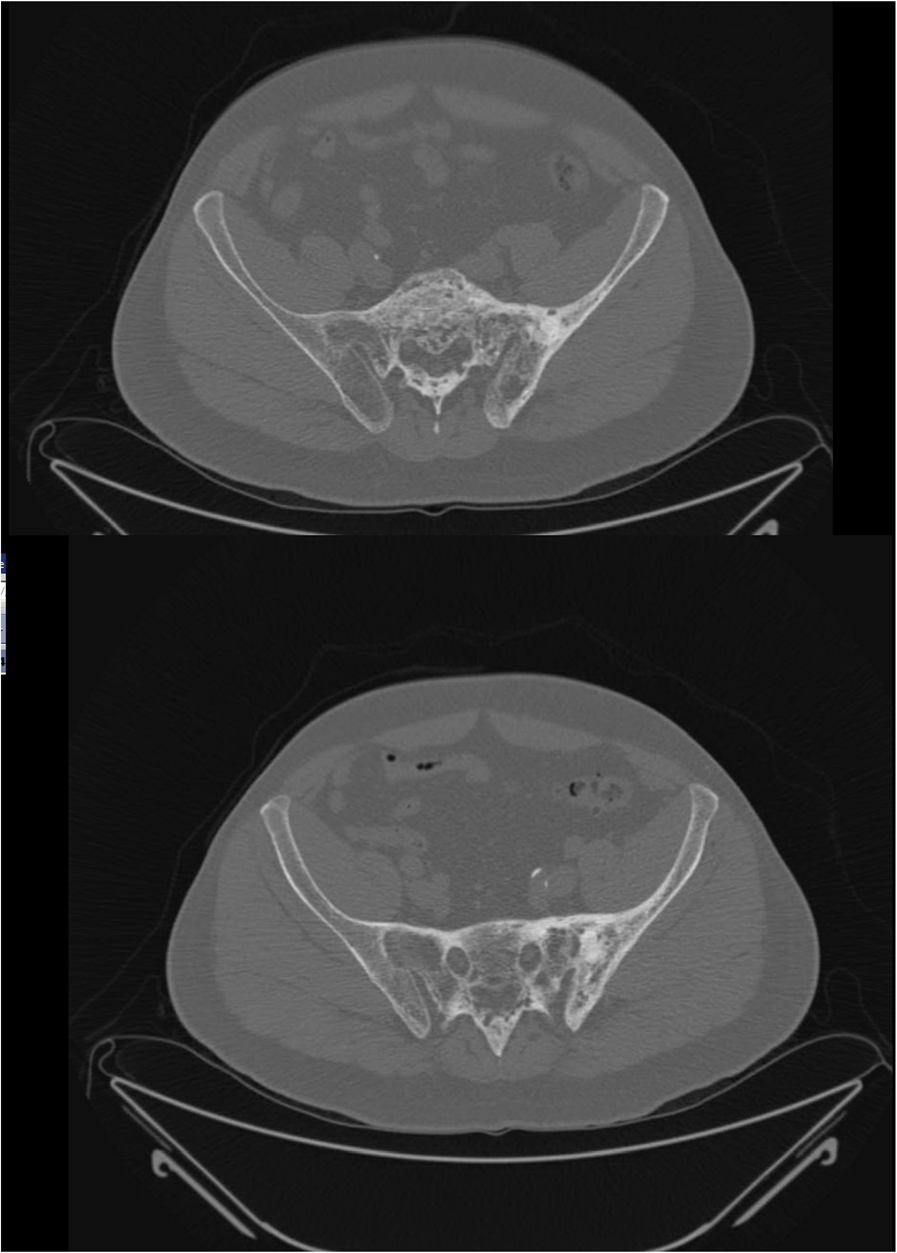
CTscan of pelvis. A CT scan of the pelvis of the patient reveals that the fusion of the SI joint is superior-posteriorly and the synovial part of the joint is patent. This observation suggests that involvement of SI joint can be seen without any pagetoid changes on that side
